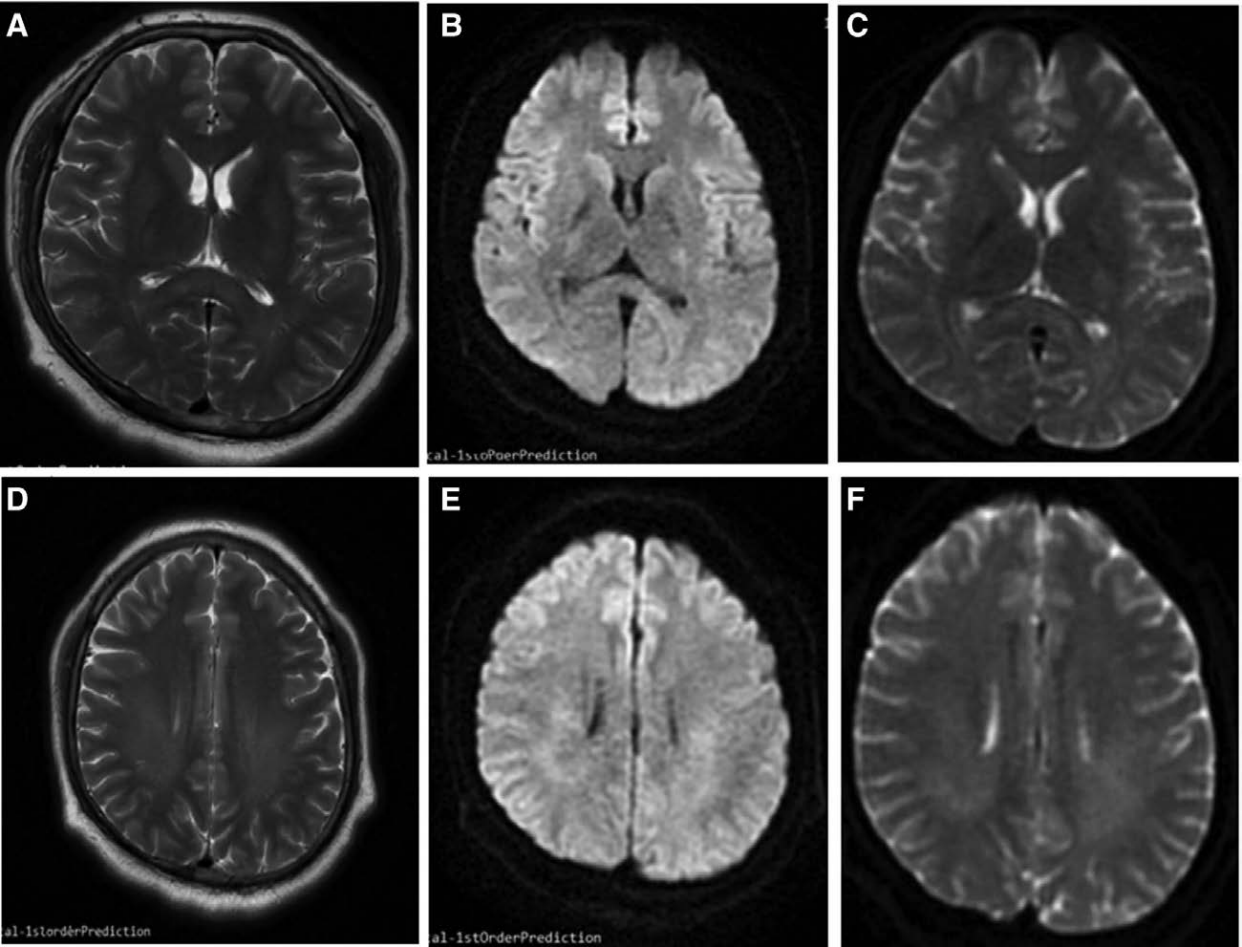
A follow-up brain MRI was performed 30 days discharged from hospital, and the lesions determined on the second MRI were significantly regressed in T2, DWI and ADC (A, B and C, D, E and F). ADC = apparent diffusion coefficient, DWI = diffusion-weighted imaging, MRI = magnetic resonance imaging.
